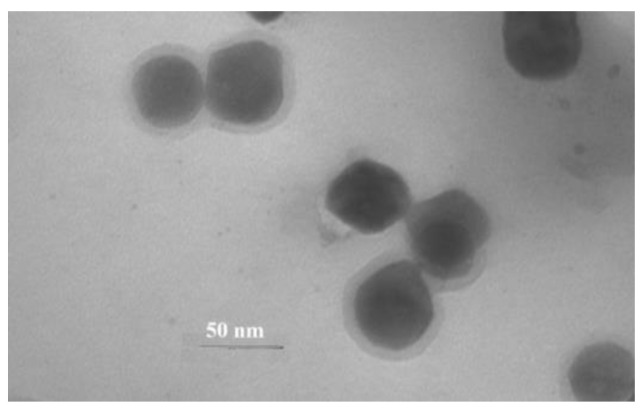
TEM image of the product of thermolysis of silver fumarate by ultrasound in an aqueous medium after 7 min of the start of treatment.
